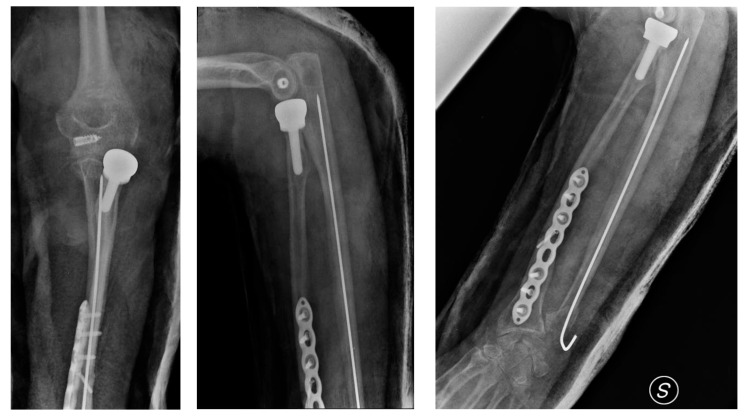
Postoperative follow-up X-rays.
